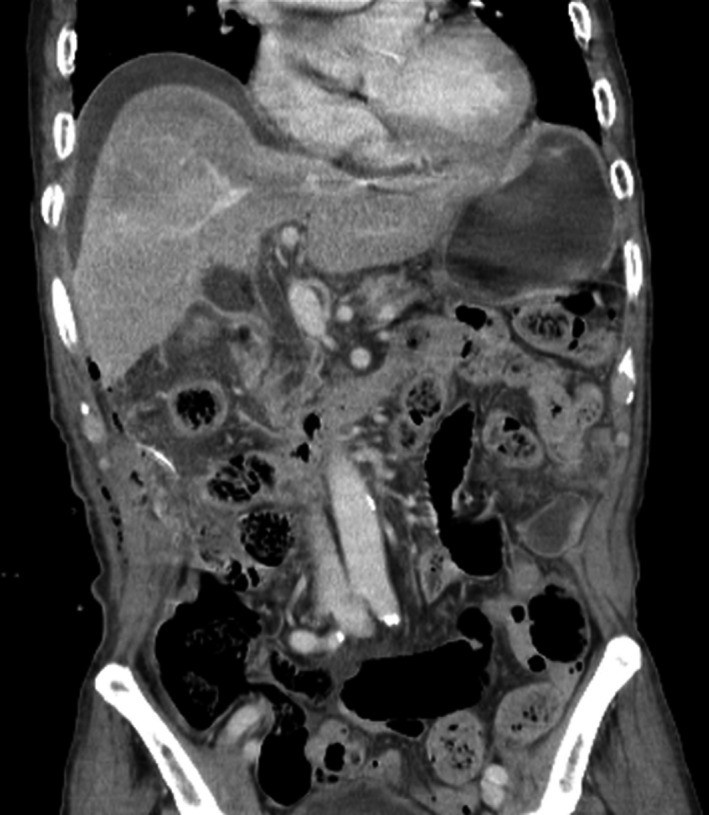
Computed Tomography (CT) Scan after Bile Leakage. CT shows pneumoperitoneum in the upper abdomen and diffuses fluid collection in the right perihepatic space
